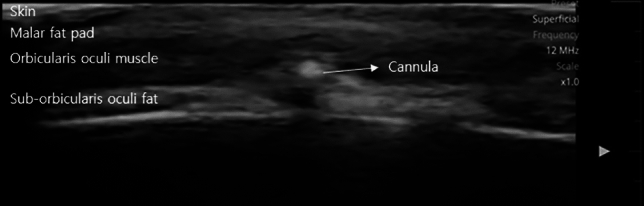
Intraoperative Ultrasonography finding demonstrate the trajectory of the cannula during its passage
